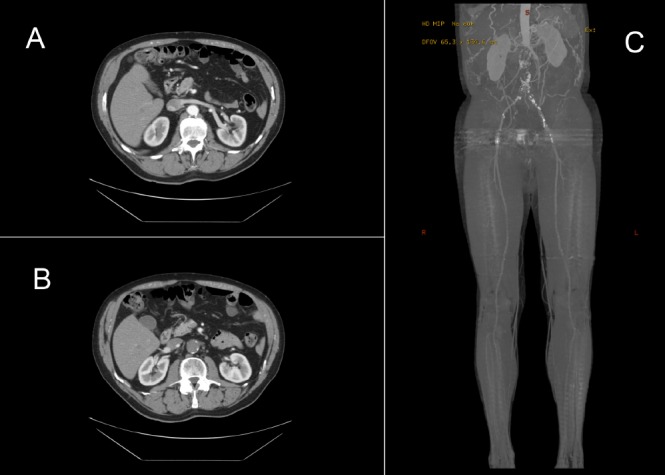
Computed tomography angiography slices with contrast showing patent aorta above renal vessels (A), complete occlusion of aorta (B) and reconstruction with complete occlusion of infrarenal aorta (C)
